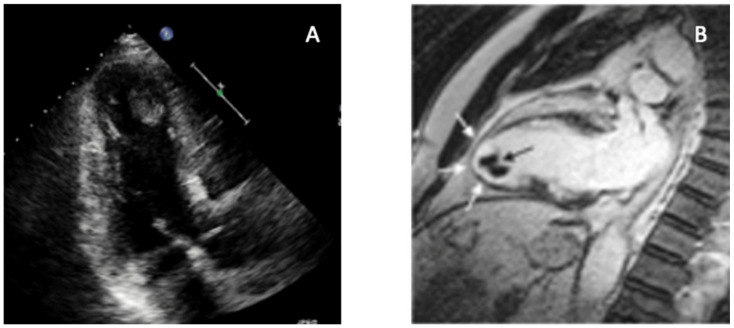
Multimodality imaging of left ventricular apical thrombus. Key teaching point: Combining contrast-poor echocardiography with CMR allows definitive differentiation between apical thrombus and other causes of apical obliteration such as ApHCM, EMF, or LVNC. (A) Transthoracic echocardiography (apical four-chamber view) demonstrates a well-defined, hyperechoic mass adherent to the LV apex, associated with severe regional wall-motion abnormality. (B) CMR cine imaging confirms an apical thrombus (black arrow), visualized as a non-enhancing, low-signal mass sharply distinct from the surrounding dyskinetic myocardium (white arrows). Multimodality evaluation enables confident identification of apical LVT and prevents misdiagnosis as hypertrophic, infiltrative, or fibrotic apical disease, directly guiding anticoagulation and clinical management. Original image from our institution (single patient).
